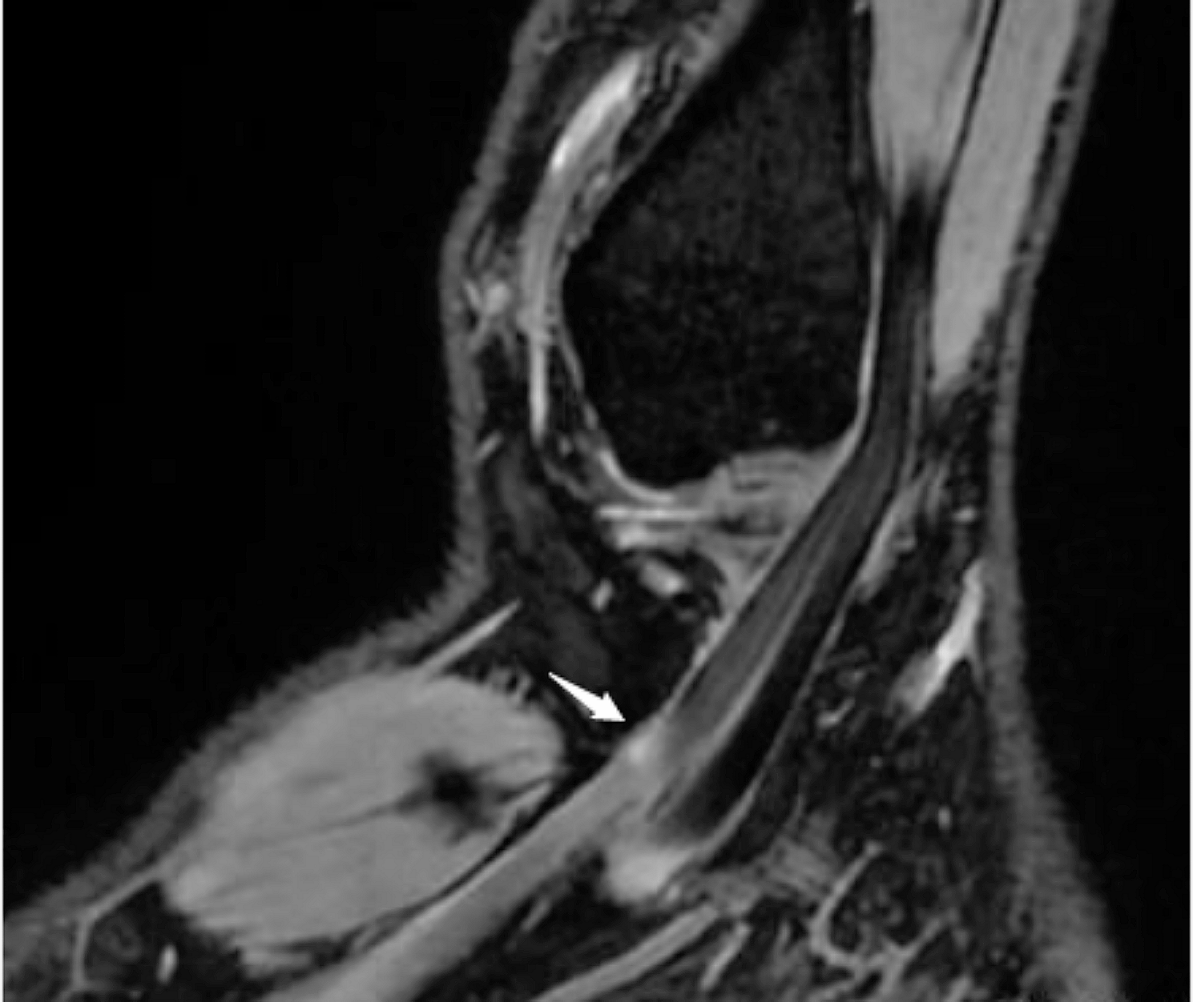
This image shows an MRI of a volunteer with a flexor halluics longus (FHL) tendon injury(Increased signal in the tendon indicated by the arrow, suggesting tendon injury)
